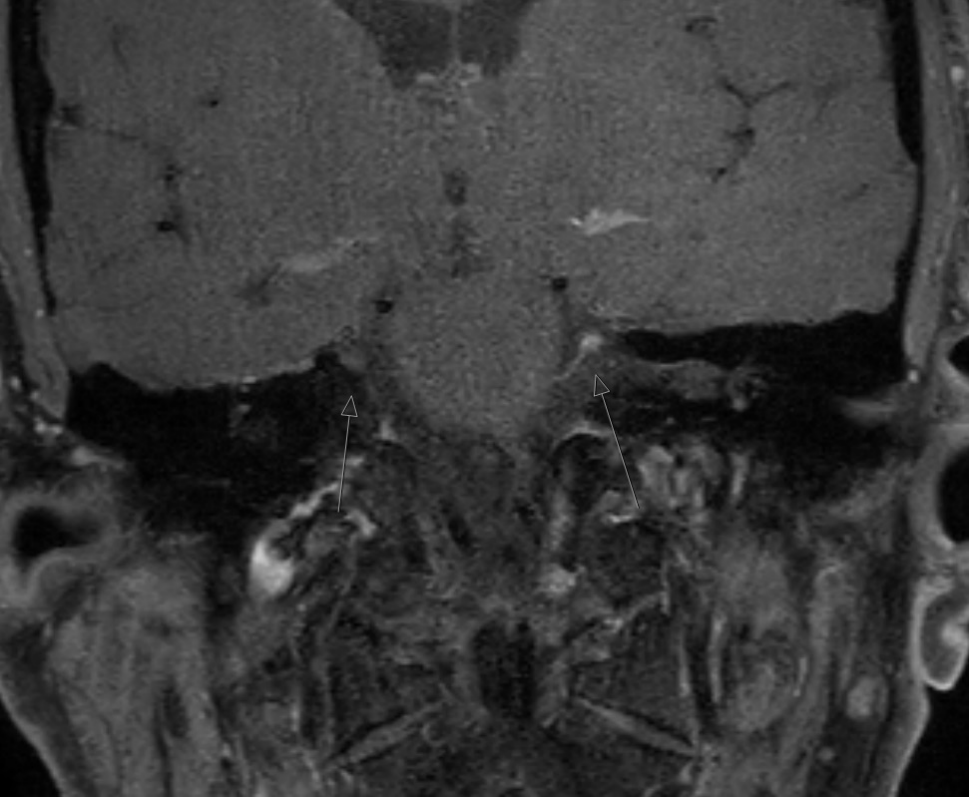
Postprocedural MRI of the brain showing enhanced signaling on the procedural side. The arrows point to the location of enhanced signalling (ie the hematoma) of the MRI.
